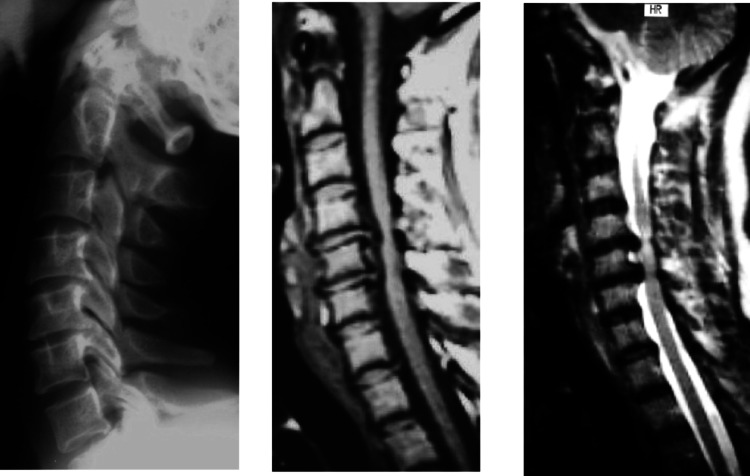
Preoperative lateral cervical spine X-ray showing C4–C5 and C5–C6 narrowed disc spaces with prominent posterior osteophytes (a). Sagittal T1- and T2-weighted MRI images demonstrating a disc/osteophyte complex causing significant spinal cord compression with intramedullary signal changes (b, c)
